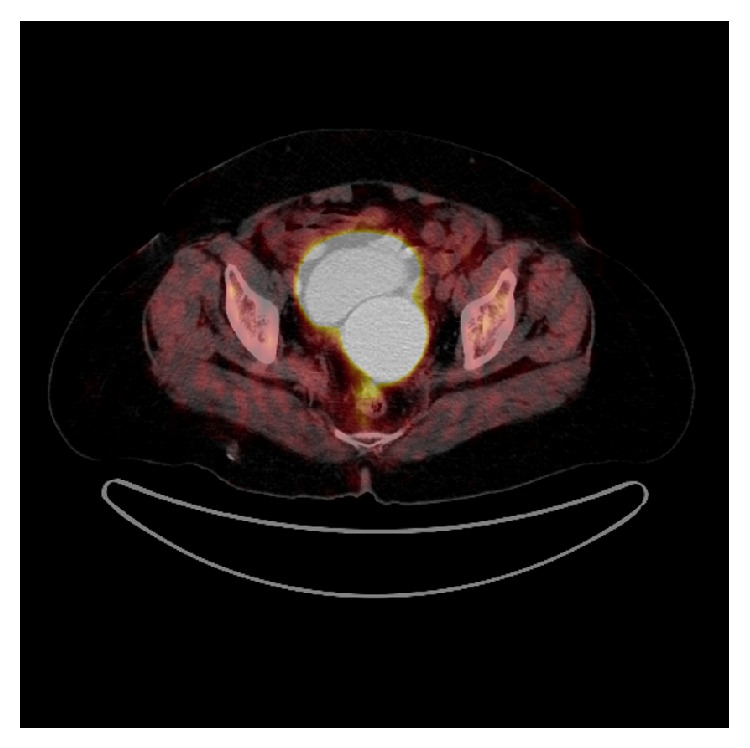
PET-CT demonstrates a mass of malignant melanoma in size of 65 × 63 × 98 mm with the uptake of fluoro-2-deoxy-D-glucose (FDG) (suv MAX 27.37) in uterine cervix area.
